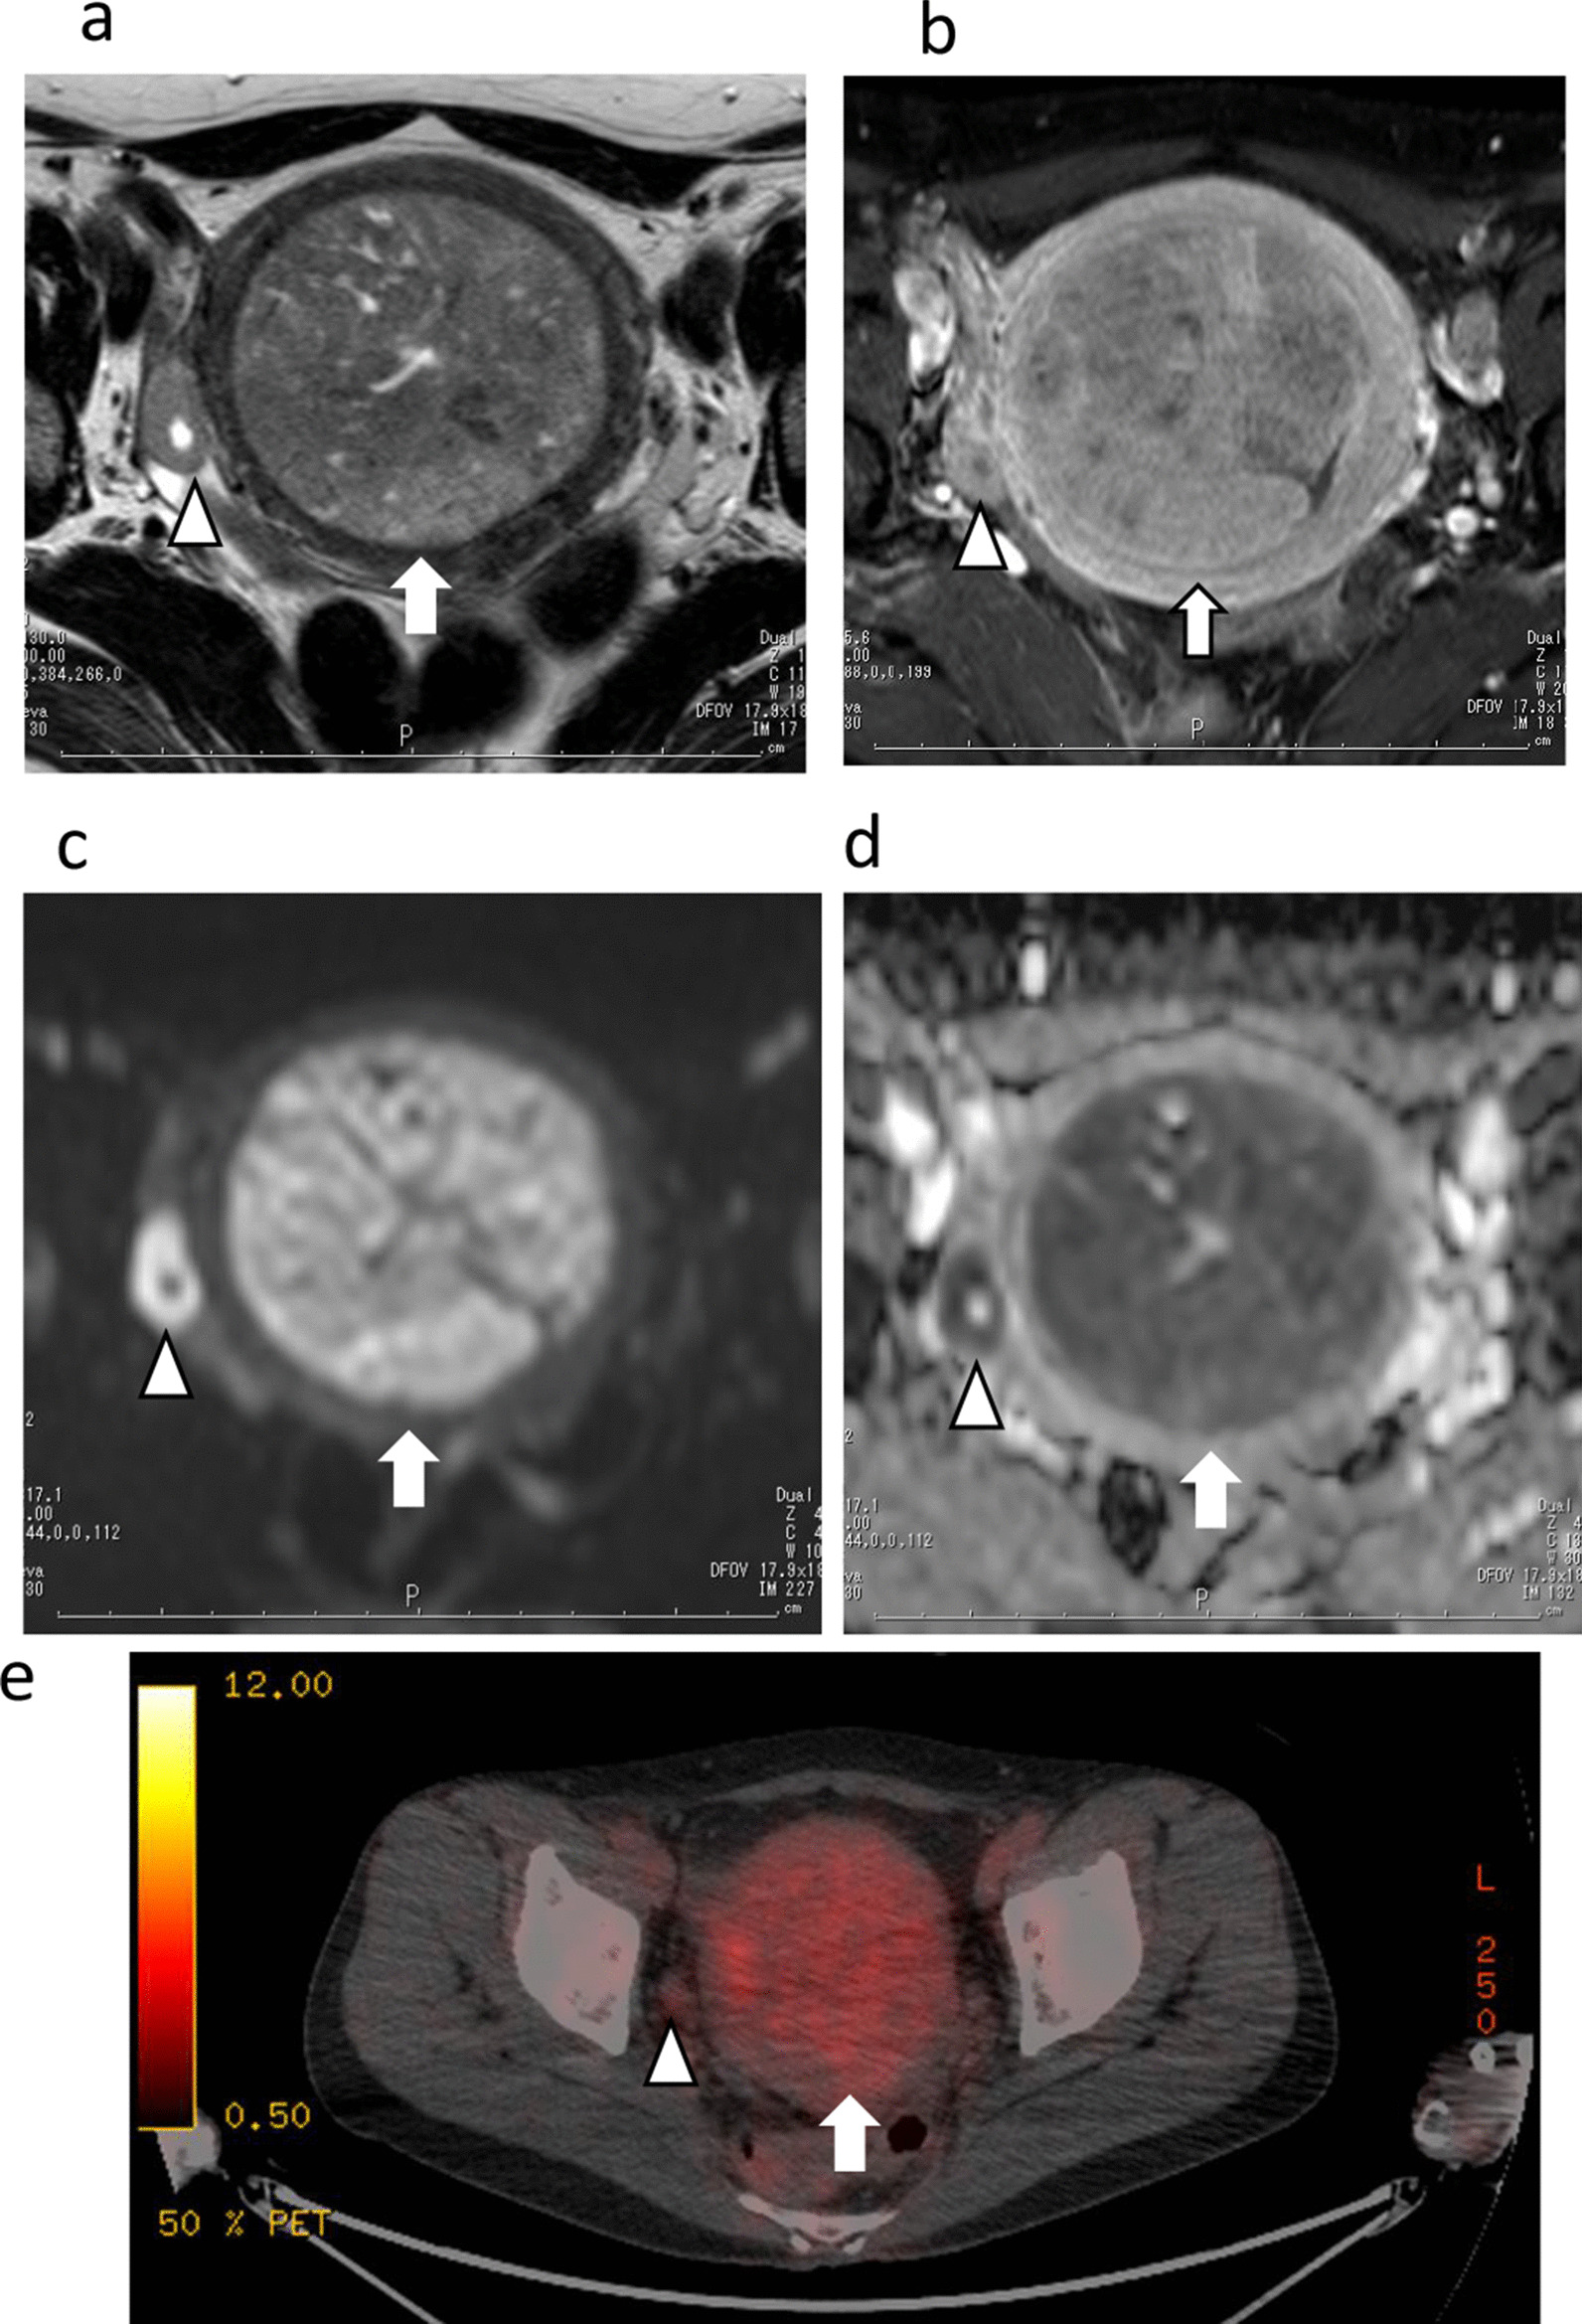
(a) Axial T2 weighted imaging (TR/TE, 6130/100 ms) showed an intrauterine mass (arrow) with clearly defined inhomogeneous high intensity. (b) The mass (arrow) showed inhomogeneous contrast enhancement with cystic changes of variable sizes on post-contrast fat-suppressed T1 weighted imaging (TR/TE, 575/13 ms), high signal intensity on diffusion-weighted imaging (DWI) (b = 1000 s/mm^2^, TR/TE, 4317/70 ms) (c) and low signal intensity (0.88 × 10^−3^ mm^2^/s) on apparent diffusion coefficient (ADC) maps (d). A 22-mm solid nodule accompanied by a tiny cyst was detected in the right ovary (a–d arrowhead). The nodule showed homogenous iso signal intensity with the uterine tumor on T2WI (a). It showed homogeneous contrast enhancement with a tiny cystic change (b), marked high signal intensity on DWI (c) and low signal intensity (0.66 × 10^−3^ mm^2^/s) on ADC maps (d). Whole-body 18F-FDG positron emission tomography-computed tomography (PET-CT) showed increased FDG uptake (maximum standardized uptake value: 8.2) in the uterine tumor (arrow) and no abnormal FDG uptake in any other organs, including the right ovarian nodule (e arrowhead)
